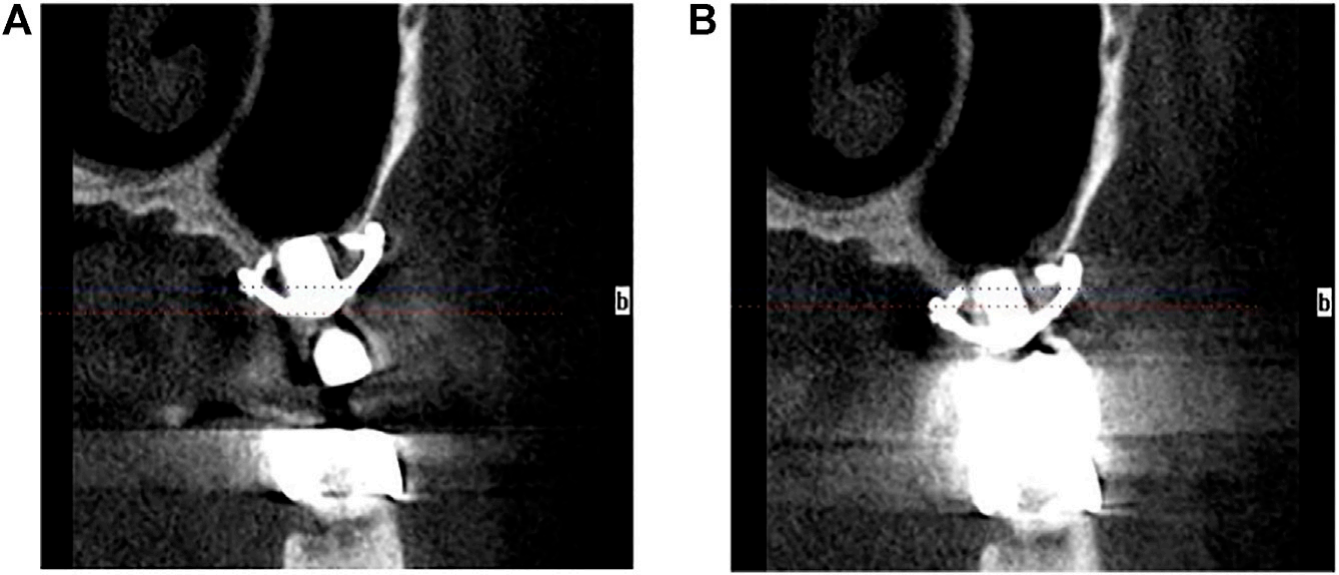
The CBCT images. (A) 6-month postoperative CBCT image. (B) 12-month postoperative CBCT image.
